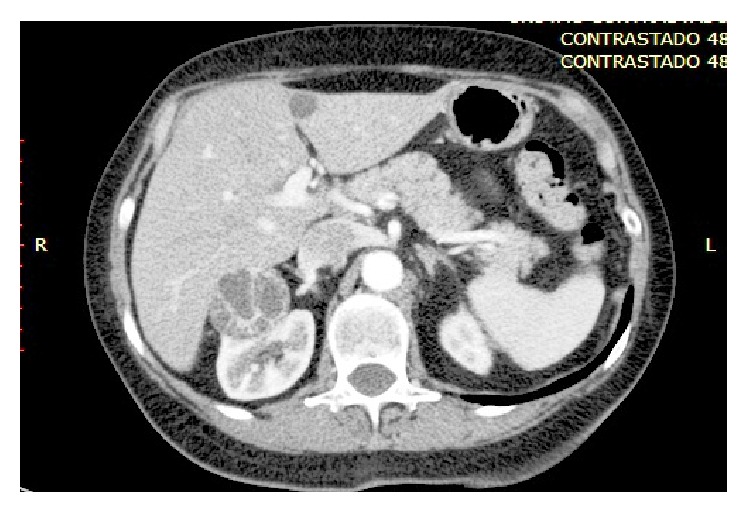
Contrast-enhanced CT scan (corticomedullary phase): complex cyst compatible with a Bosniak III cystic renal mass.
